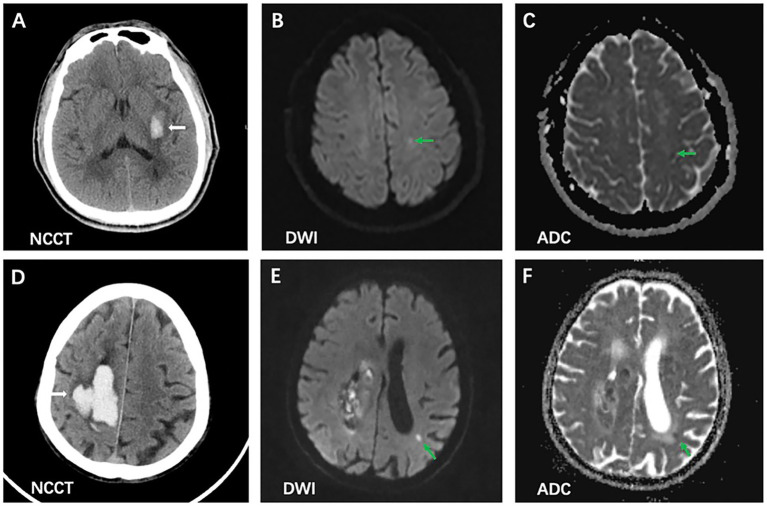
Remote DWI lesions in ICH. (A–C) Hypertensive ICH. Left basal ganglia hemorrhage, small punctate DWI hyperintensity in left parietal lobe with corresponding hypointensity in apparent diffusion coefficient (ADC) map. (D–F) Probable cerebral amyloid angiopathy-related ICH. Right parietal lobe hemorrhage, small punctate DWI hyperintensity next to the posterior horn of the left lateral ventricle with corresponding hypointensity in ADC map. NCCT, non-contrast computed tomography; DWI, diffusion-weighted imaging; ADC, apparent diffusion coefficient; ICH, intracerebral hemorrhage; white arrows indicate ICH lesions, green arrows indicate remote DWI lesions.
